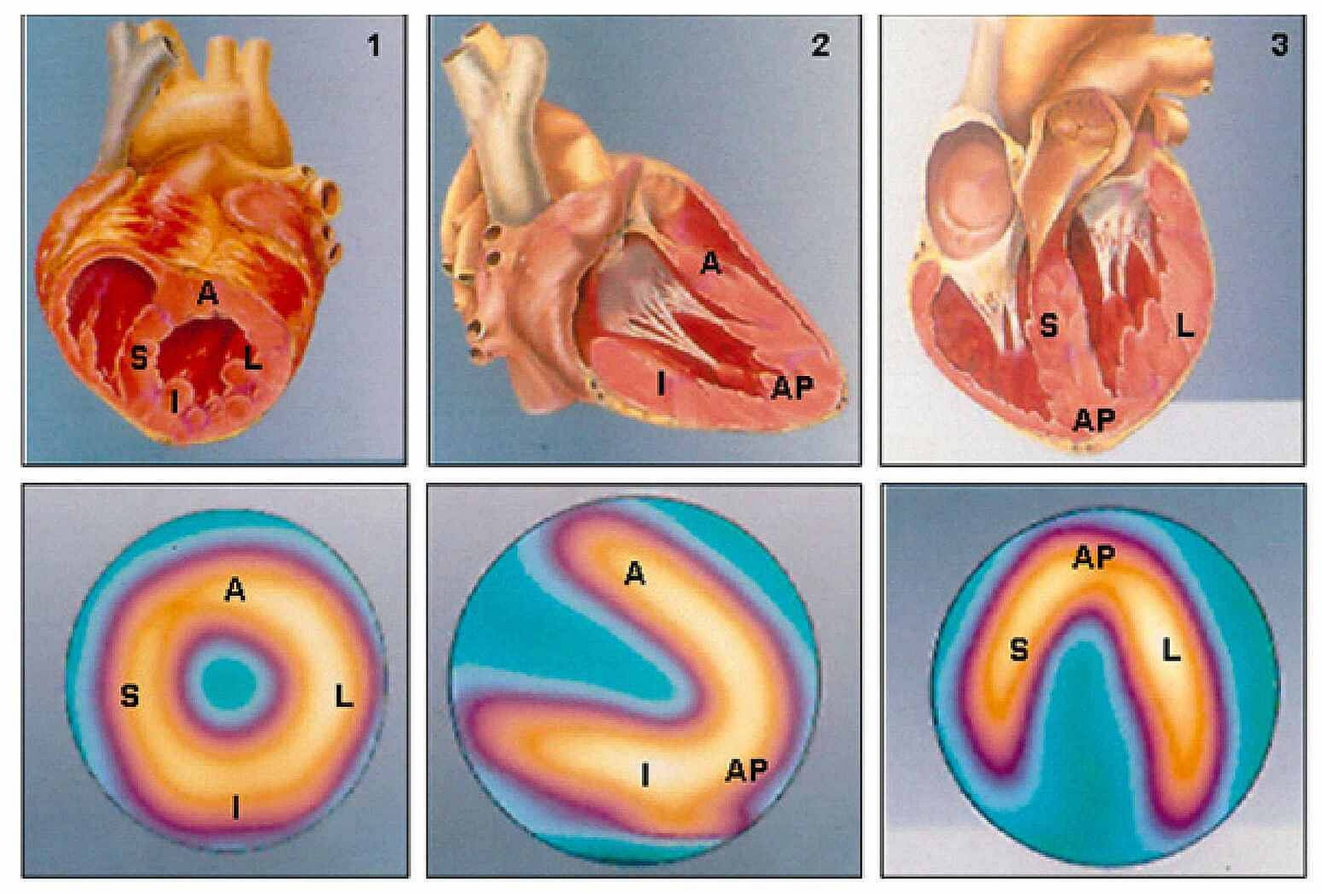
Two-dimensional reconstructive images of scintigraphy (lower images) and its correspondence to three-dimensional heart model (upper images) Two-dimensional reconstruction of scintigraphy images representing normal perfusion patterns (lower images), in line with the minor axis (1), vertical long axis (2), and horizontal long axis (3) cross-sections and their respective corresponding anatomical cross-sections (upper images) A: anterior; AP: apical; I: inferior; L: lateral; S: septal Adapted from Mastrocola LE [24]
